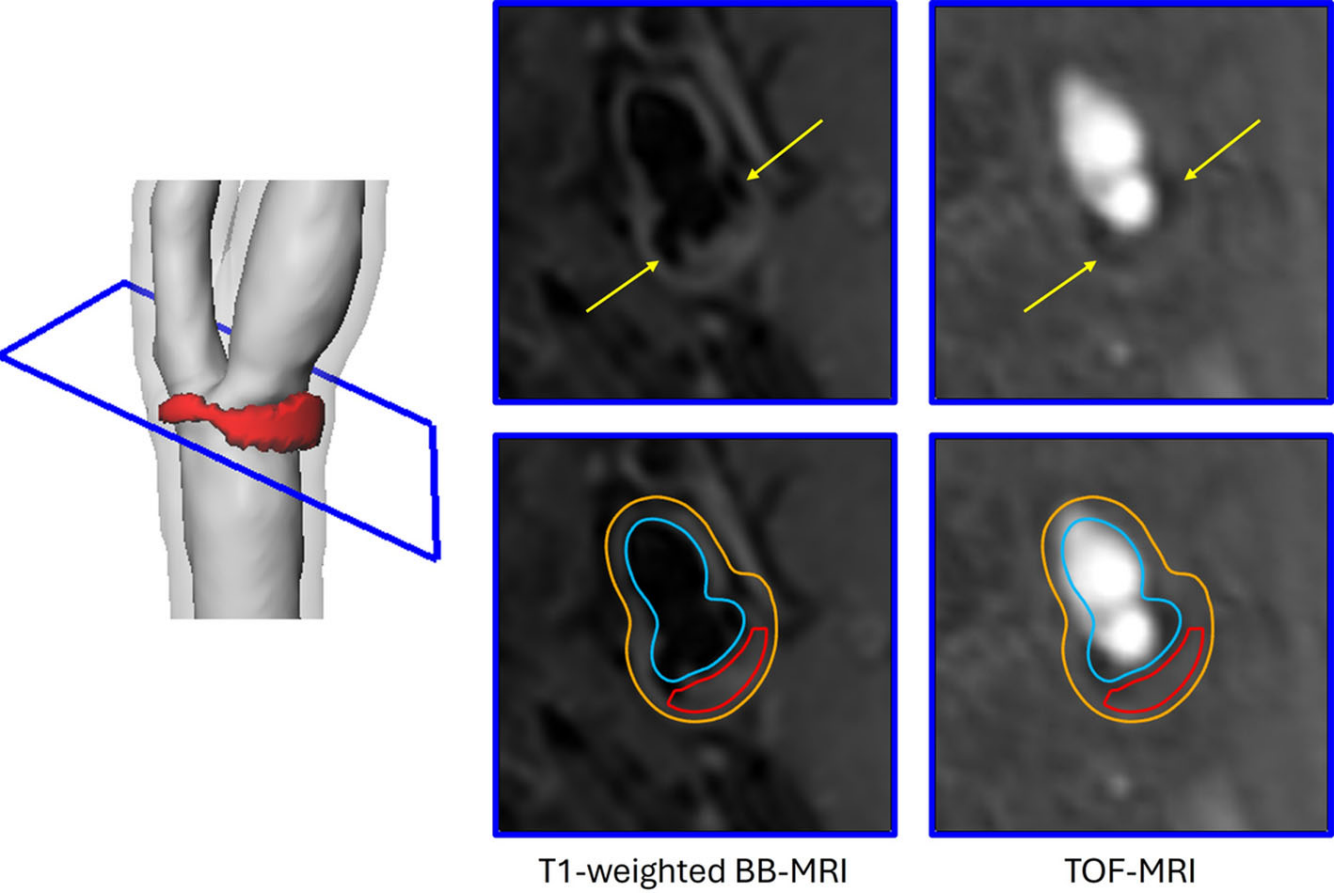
Cross section of the T1-weighted BB-MRI and TOF-MRI of the follow-up examination in Fig. 4c. The outer vessel wall (orange), inner vessel wall (blue), and lesion region (red) show that the 3D segmentation failed in this case, as the hypo-intense areas (yellow arrow) are not part of the lumen but are calcifications and part of the vessel wall and lesion
